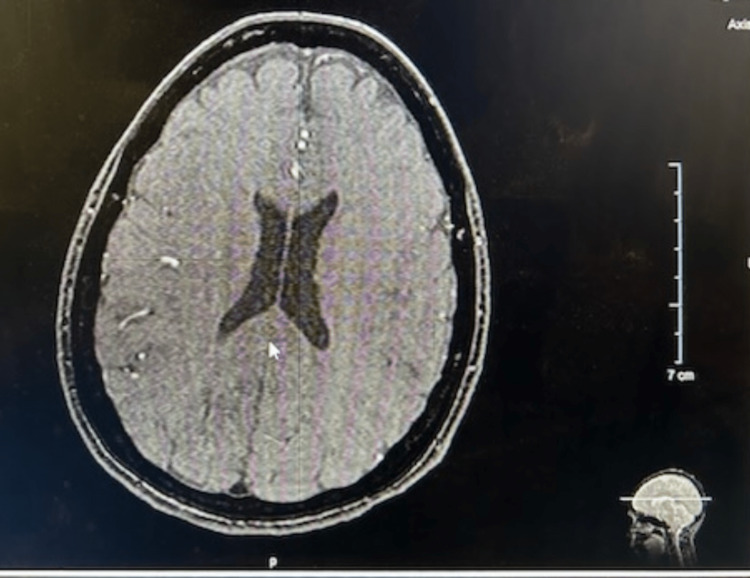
The MRI of the brain (axial view) showed no relevant or significant changes
